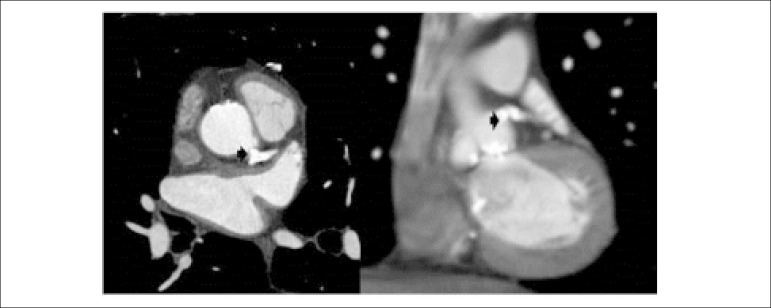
Cardiac CT. Pre-TAVI cardiac CT showing heavy calcification (arrow) extending into the left main ostium, left anterior descending artery and left circumflex artery as well as annular calcium.
